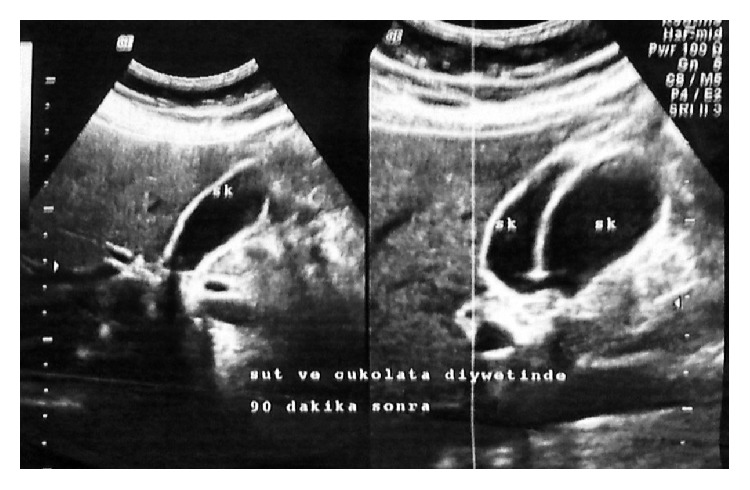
Ultrasound image of both gallbladders.
